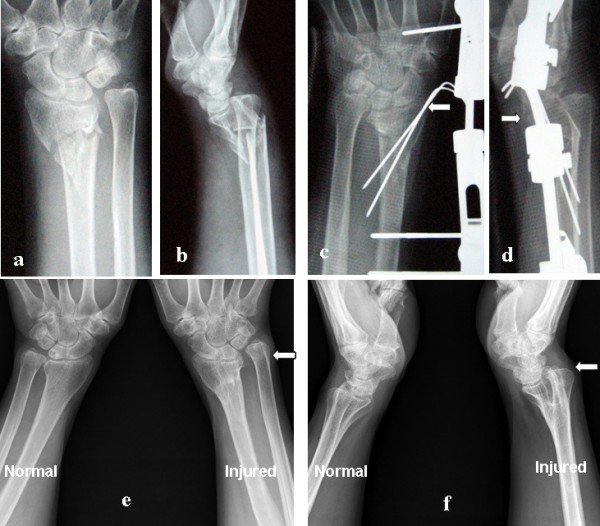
The 56-year-old female patient with unstable distal radius fracture. (a, b) The initial anterior-posterior and lateral radiographs of the distal radius showed an unstable fracture without an ulnar styloid fracture: volar comminution of the metaphysis. (c, d) Three weeks postoperatively, the fracture segments were displaced (white arrow); the patient declined re-operation and her wrist was fixed with above-the-elbow plaster. (e, f) Two years postoperatively, the distal radius fracture had mal-united with radius shortening and instability of the distal radioulnar joint (white arrow). Written informed consent was obtained from the patient to show the information here.
